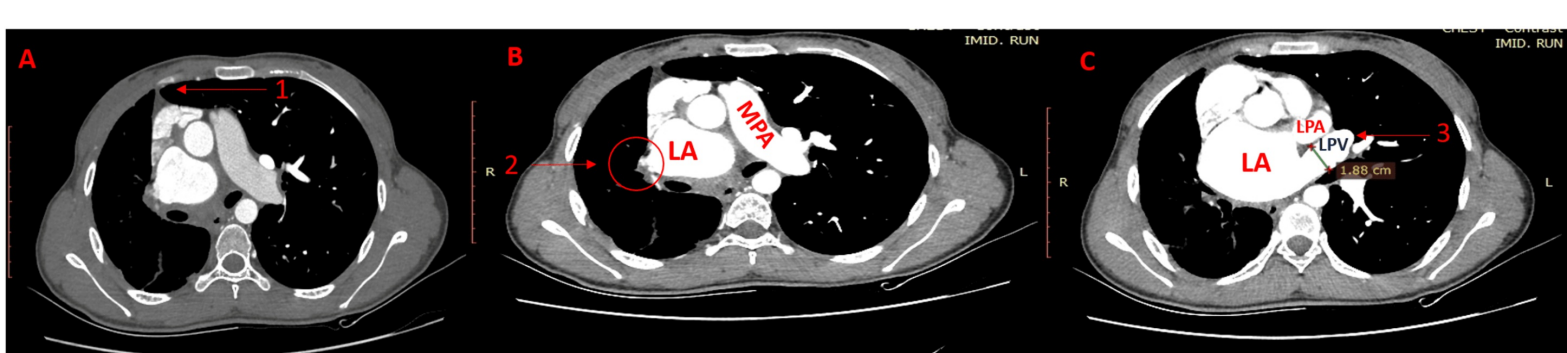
Contrast-enhanced CT scan (A) Herniation of the left lung (1). (B) Reduced caliber of the right pulmonary vein (2), dilated left atrium (LA), and main pulmonary artery (MPA). (C) Dilated left pulmonary vein (LPV) (3), dilated left atrium (LA), and left pulmonary artery (LPA).
